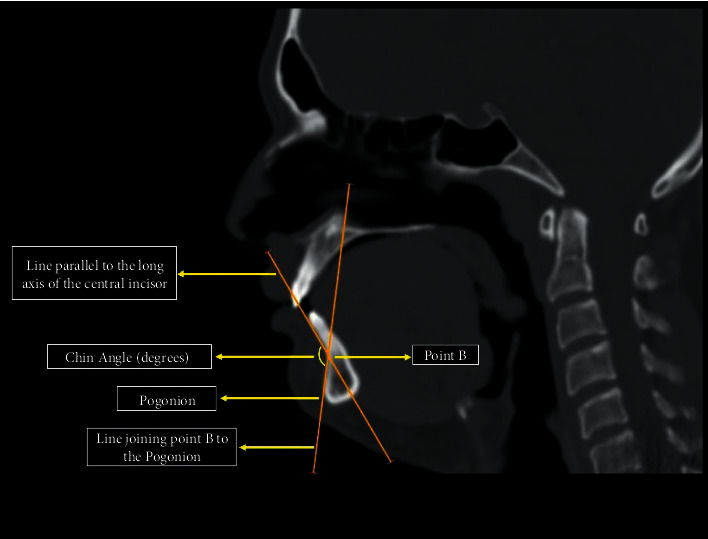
The chin angle depicted in the midsagittal CT scan.
